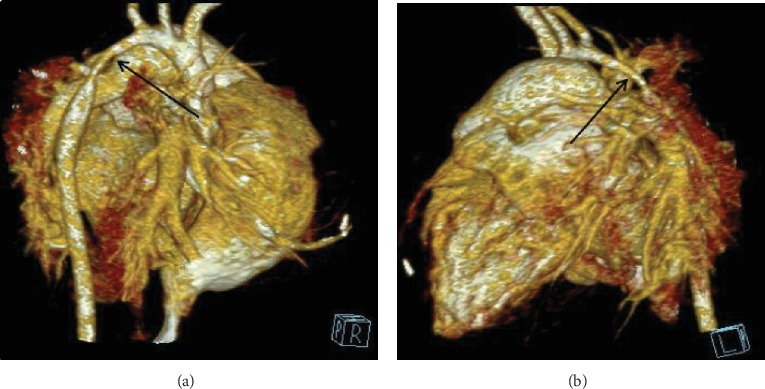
Classic CTA images: arrows highlight the aortic coarctation (COA).
